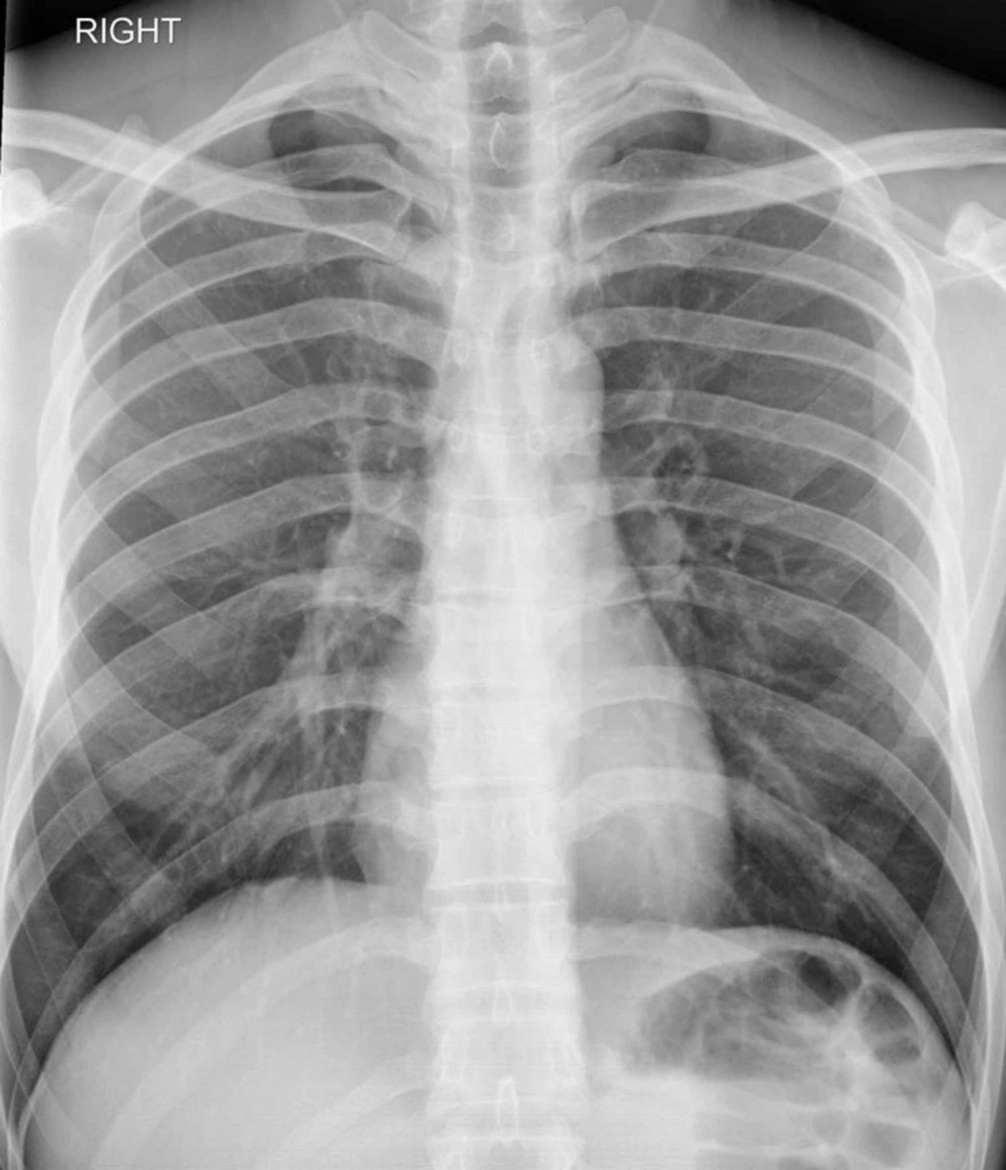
X-ray chest AP view showing normal lung fields AP, anteroposterior
